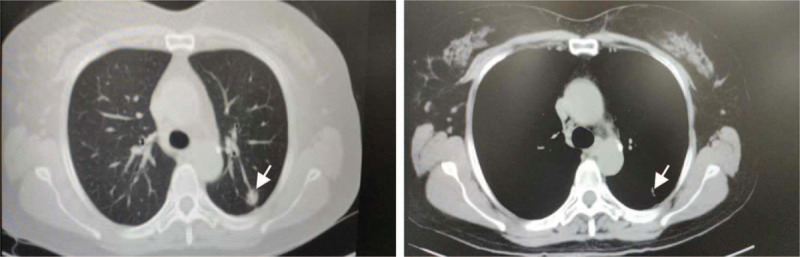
Computed tomography (CT) scan revealed a tumor mass in the upper lobe of left lung (arrow).
